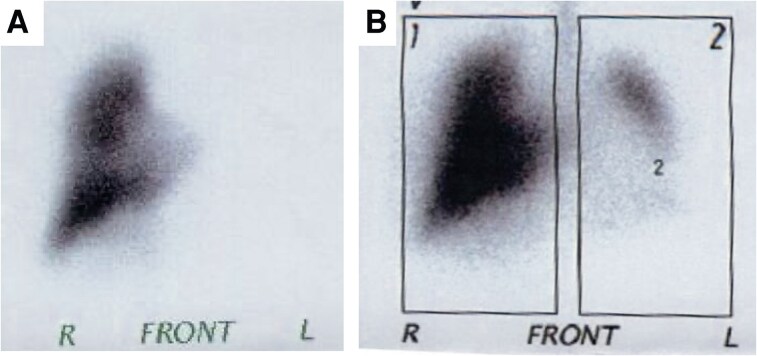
Lung scintigraphy showing no perfusion (*A*) and decreased ventilation (*B*) of the left lung.
